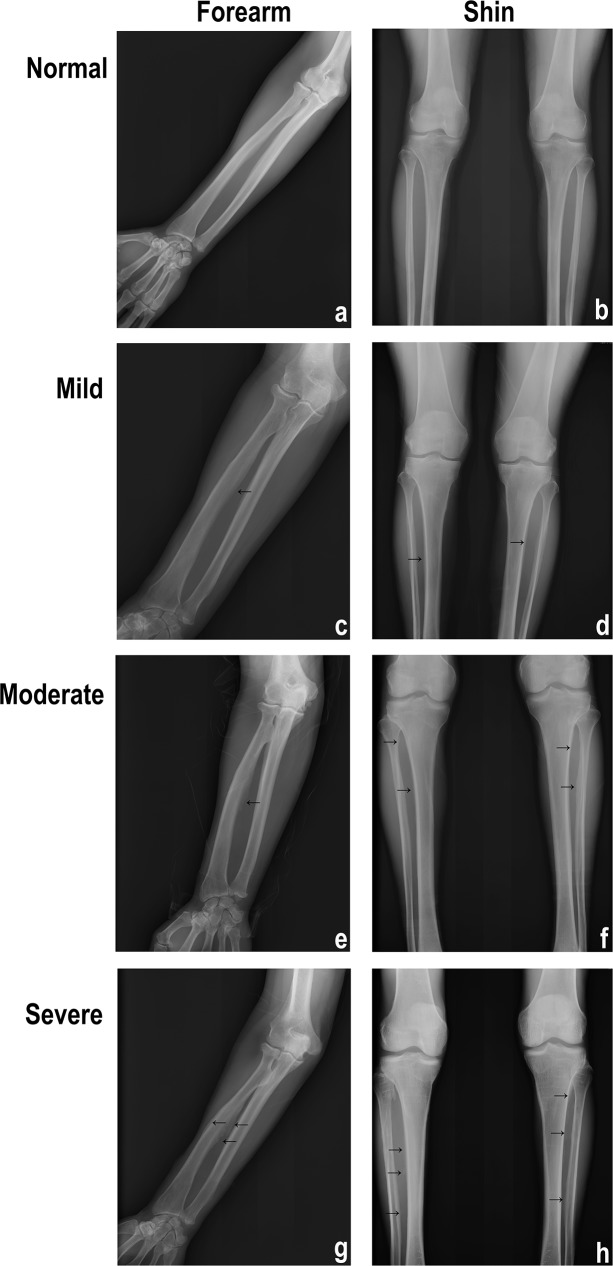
Representative images of brick-tea type skeletal fluorosis by X-ray examination. Normal (a) and (b): Normal forearm and shin. Mild (c) and (d): The interosseous membrane in the forearm or lower leg bone was mildly ossified with rough edges (arrows). Moderate (e) and (f): The radial crest and the border were enlarged; the interosseous membrane in the forearm or shin was ossified with serration or wave edge (arrows). Severe (g) and (h): The radial crest was enlarged and the border was obviously enlarged so that ulna and elbow joints presented severe degenerative changes; the interosseous membrane in the forearm or shin was obviously ossified with large shape (arrows).
